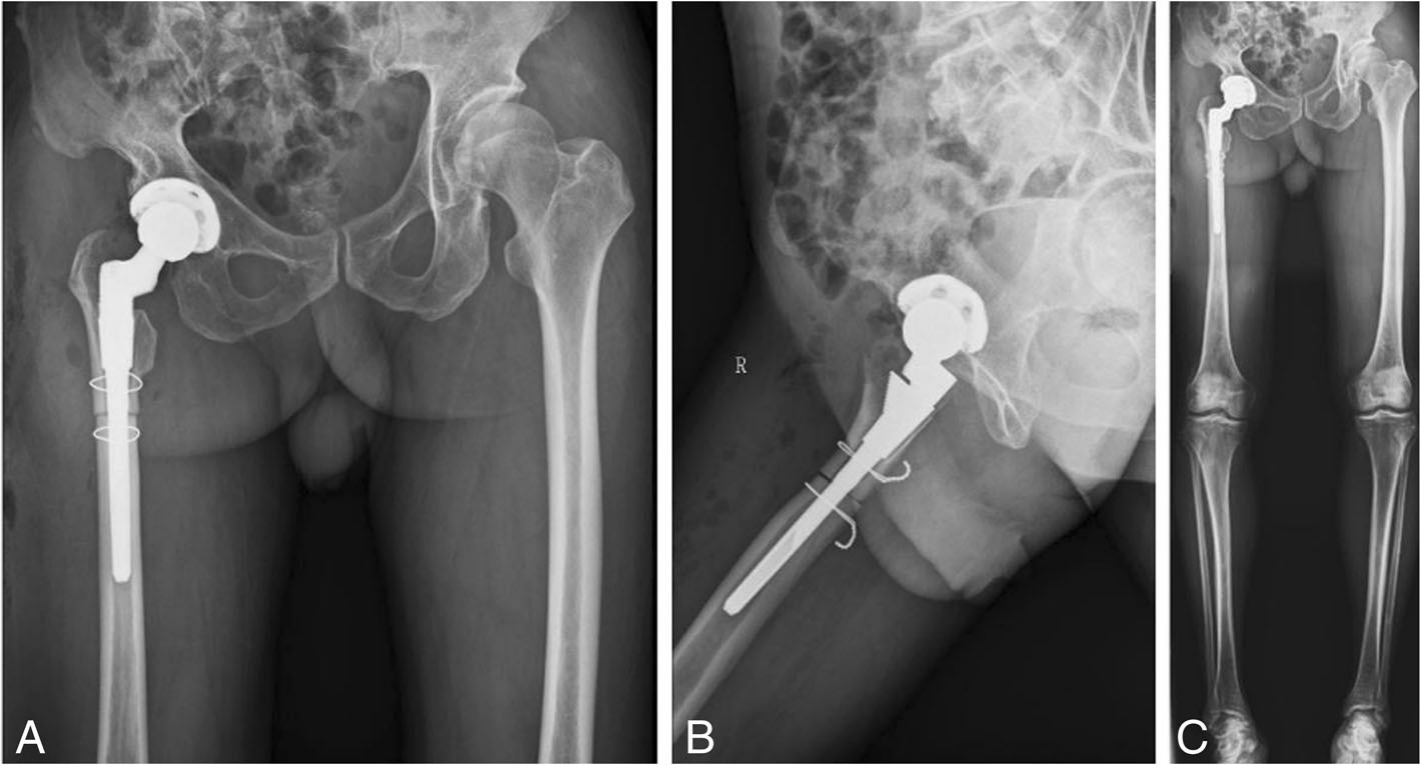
Radiographs of both lower extremities and the right hip after the operation. (a) anteroposterior view of the right hip, (b) lateral view of the right hip, (c) anteroposterior view of both lower extremities
